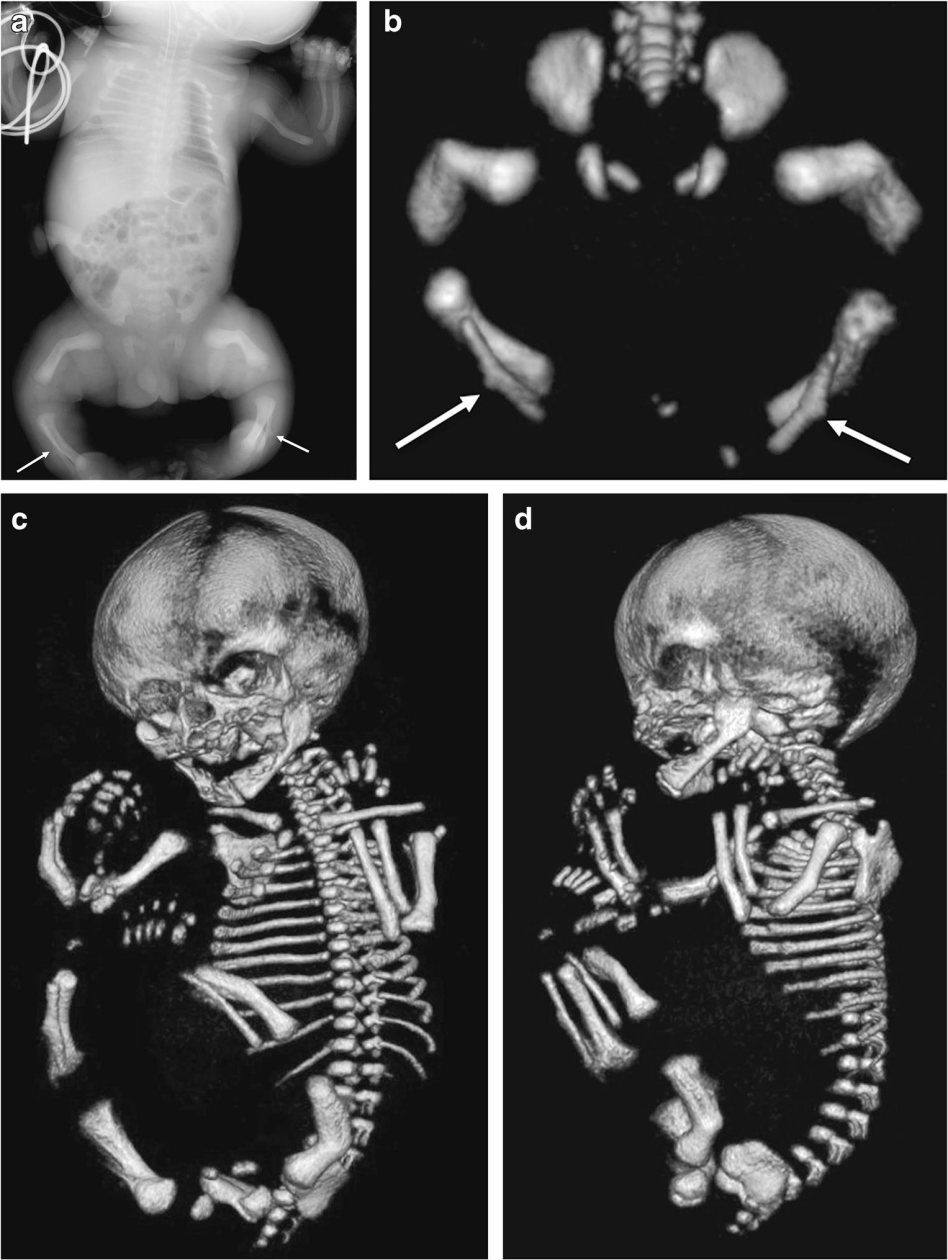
Images of a male of unknown age with benign perinatal hypophosphatasia show a less aggressive phenotype*.*a,b Anteroposterior radiograph (a) and three-dimensional reconstructed CT of the lower limbs (b) show bowing of the long bones but normal metaphyses; note the Bowdler spurs of the fibulae (*arrows*). c,d Three-dimensional whole-body CT images show skull vault ossification that is within normal limits
